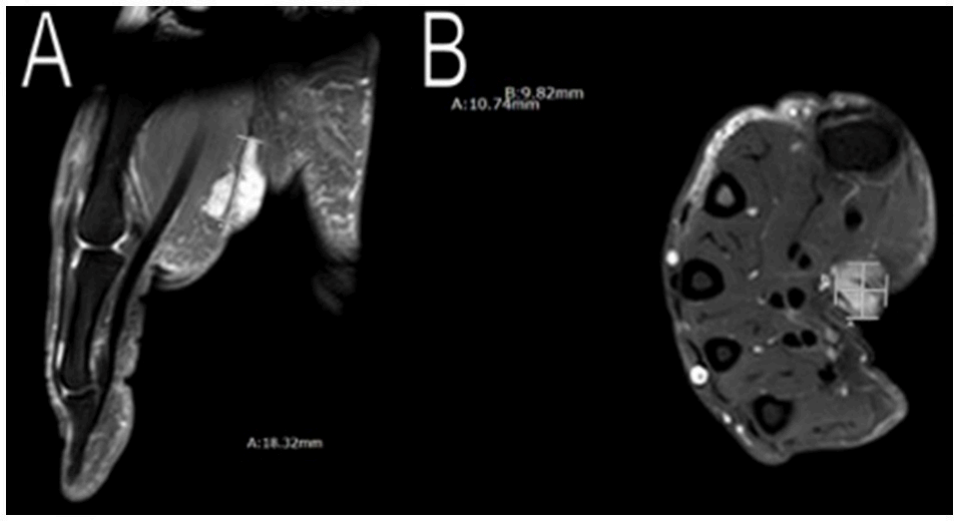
Imaging of her right palm mass. MRI scans showed an approximately 11 × 10 × 20 mm mass in her right palm. Plastic surgery excised the mass and pathology confirmed a benign nerve sheath tumor, consistent with Schwannoma (A,B).
